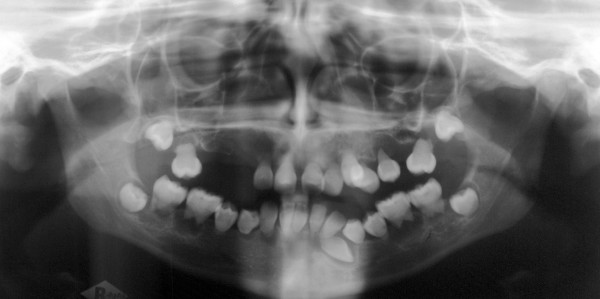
preoperative panoramic radiographs showing features of dentin dysplasia type I.
